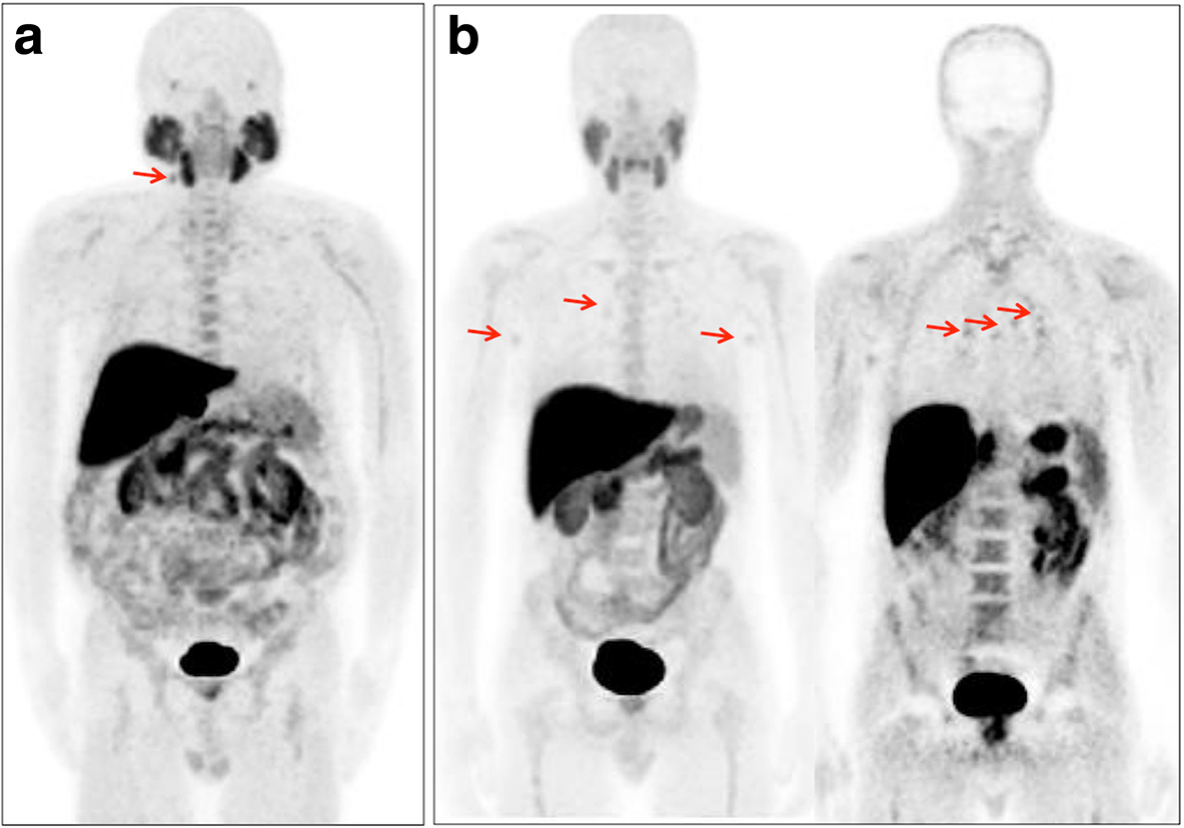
Examples of pitfalls at [^18^F]fluorocholine PET/CT in presence of inflammation. Mild [^18^F]fluorocholine uptake might be present in reactive lymph nodes as shown in these patients (not included in the present series but reported as examples) who present along with prostate lodge recurrence from prostate cancer increased [^18^F]fluorocholine uptake in reactive cervical lymph node (a, MIP arrow) and bilateral axillary and hilar/mediastinal lymph nodes (b, MIP and PET coronal arrows)
